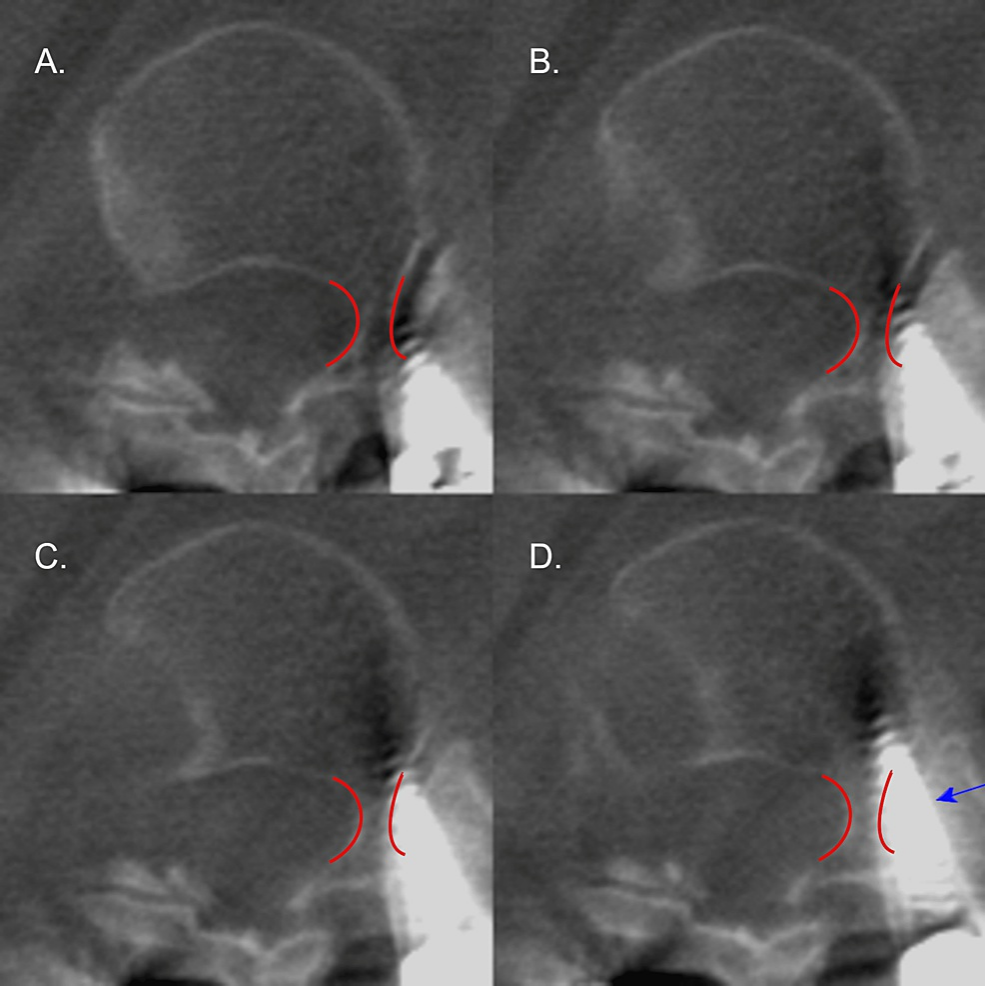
Axial CT radiographs of the left T11 pedicle screw on patient 9. Axial CT radiographs demonstrating the left T11 pedicle screw placement on patient 9 with an in-out-in (IOI) trajectory. Screw placement progresses from A to D. The pedicle of interest is outlined in red. The blue arrow denotes the largest deviation of the screw. Please note all CT images are in radiographic orientation.
